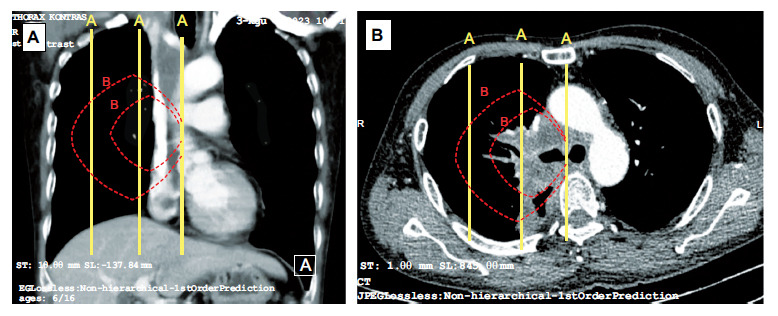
The demarcation lines for dividing the hemithorax into three-thirds using straight lines (A lines) and concentric lines (B lines). (A). CT image in the axial plane. (B). CT image in the coronal plane.
